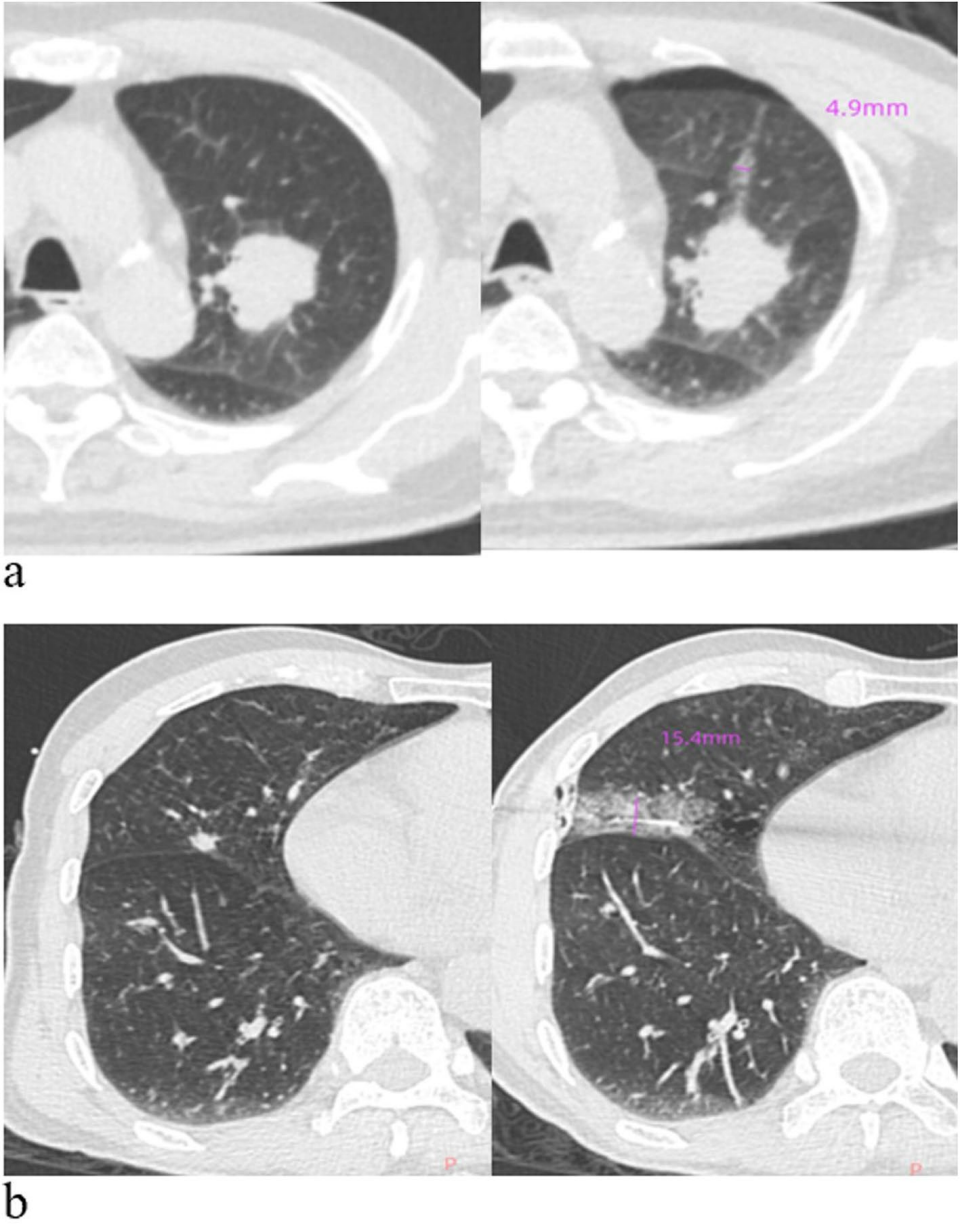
Needle track blooding (NTB) width measurement. (a) NTB with minor pneumothorax: A 4.9 mm ground-glass opacity along the needle path is seen post-biopsy after CT-PTLB on a left upper lobe mass, diagnosed as Adenocarcinoma. (b) NTB without pneumothorax: post-biopsy shows a 15.4 mm ground-glass and consolidative opacity after CT-TPLB on right middle lobe nodule, with no pneumothorax, diagnosed also as lung adenocarcinoma.
